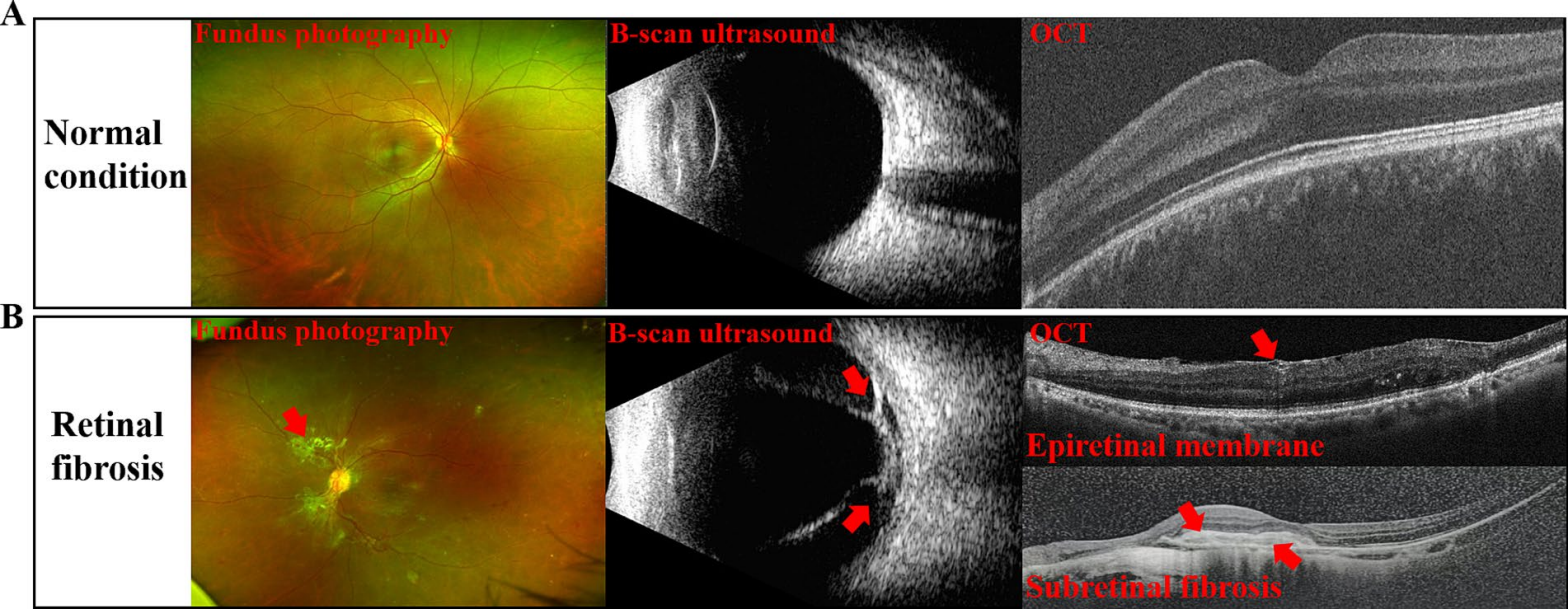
Imaging findings of retinal fibrosis. (A) Fundus photographs, B-scan ultrasound and OCT images of normal subjects; (B) Fundus photographs, B-scan ultrasound images and OCT of patients with retinal fibrosis (patients with the proliferative form of diabetic retinopathy, PDR), with the fibrotic areas indicated by red arrows.
